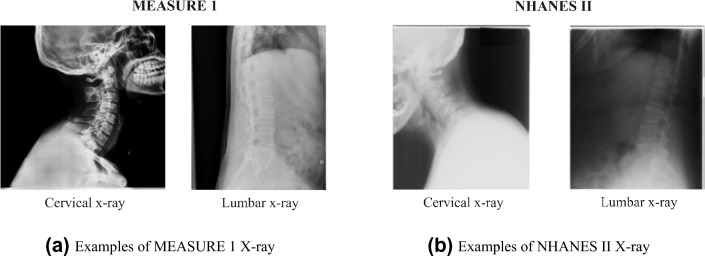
Examples of X-rays from clinical trial and public dataset; MEASURE 1 X-rays have better quality in terms of resolution, contrast, etc. in comparing with NHANES II. Quality of X-rays are similar in PREVENT and MEASURE 1.
